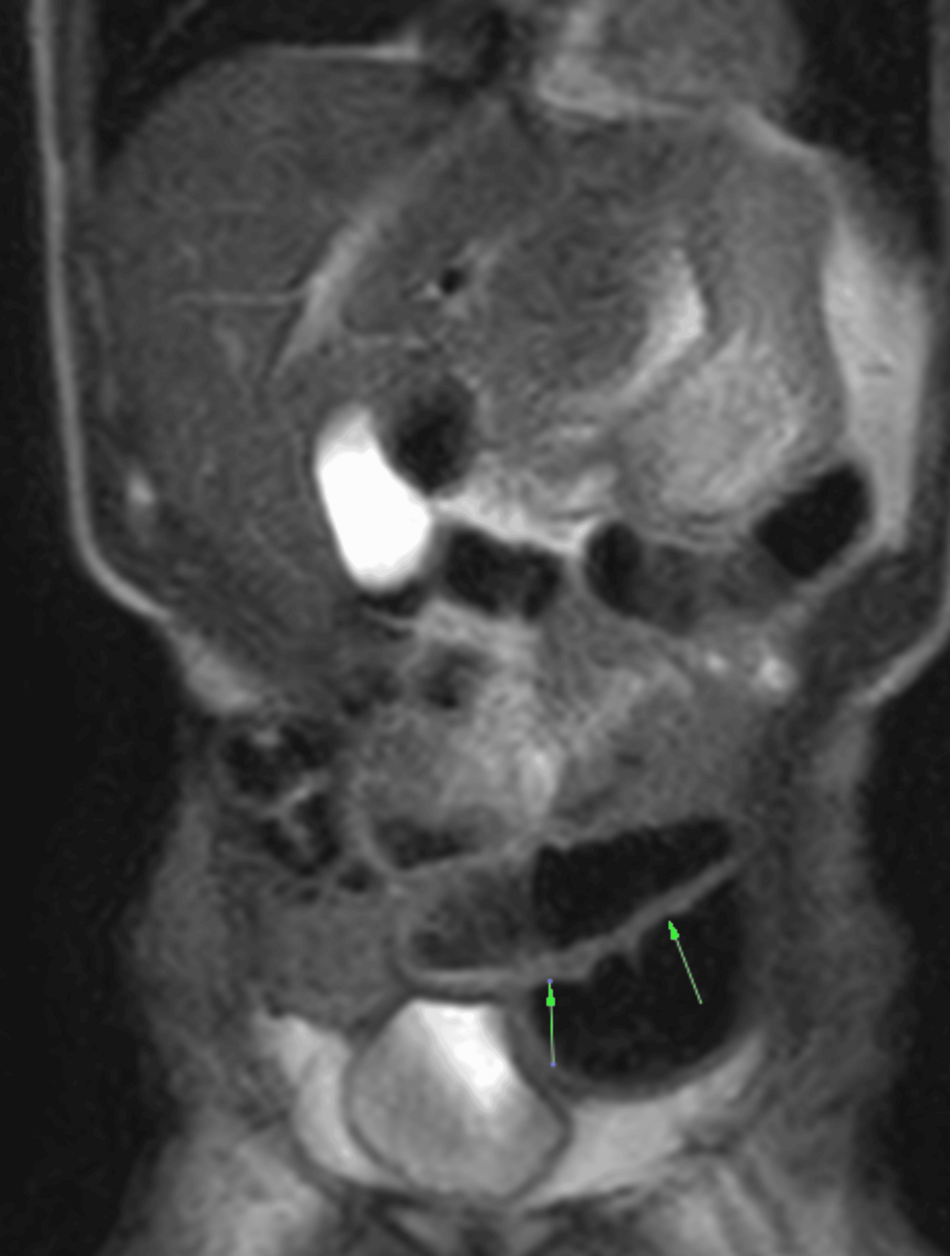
Coronal abdominal magnetic resonance imaging showing extensive ileocolonic involvement Coronal magnetic resonance image demonstrating extensive ileocolonic involvement with mural thickening and luminal narrowing, consistent with active inflammatory bowel disease (arrows).
